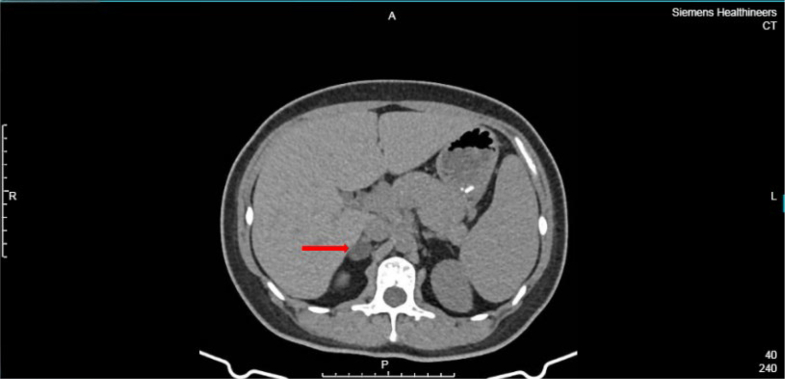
Contrast-Enhanced Adrenal Computed Tomography Scan From January 15, 2024, Revealing an Approximately 19 × 14 mm Nodule in the Right Adrenal Gland (Arrow)
